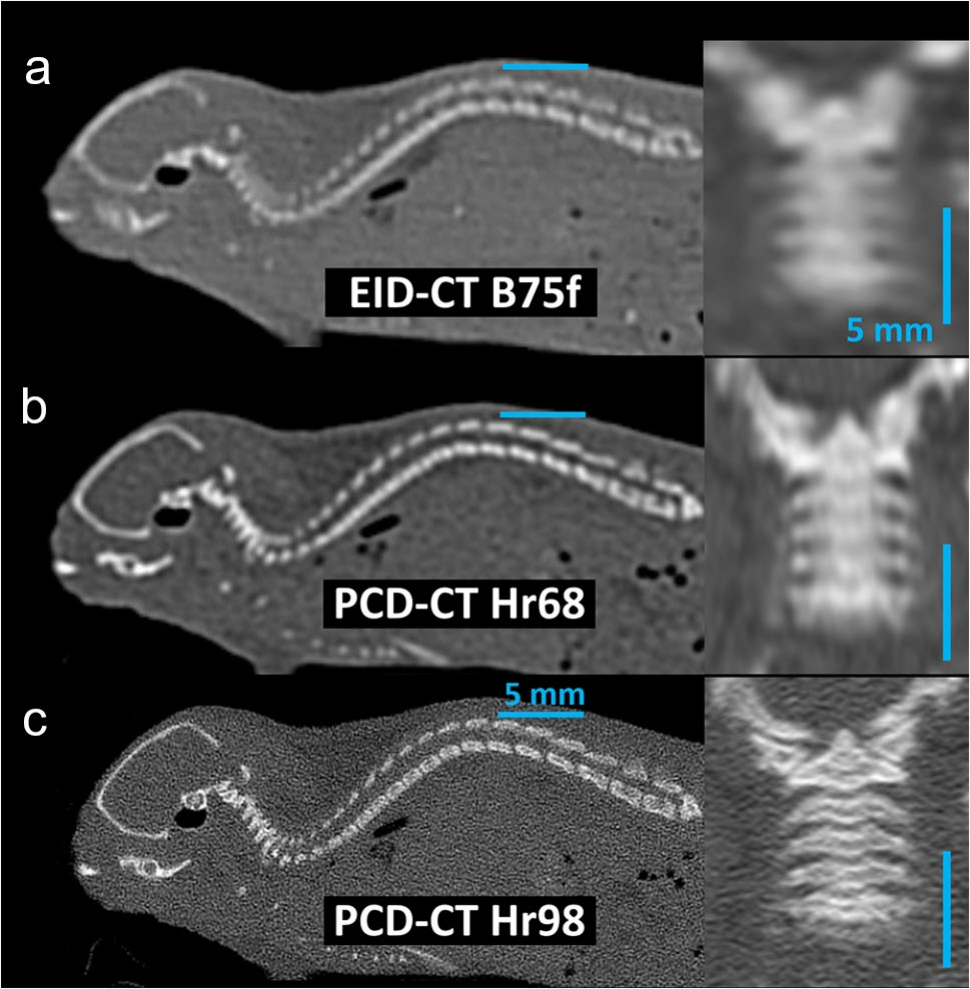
Reconstructed series used for analysis. Sagittal reconstructions of the whole spine (slice thickness: 0.2 mm) and coronal reformations of the cervical spine (slice thickness: 0.6 mm) at EID-CT B75f (a), PCD-CT Hr68 (b), and PCD-CT Hr98 (c) at CTDI_Vol_ = 15 mGy. CTDI_Vol_, volumetric CT dose index; EID-CT, energy-integrating detector CT; PCD-CT, photon-counting detector CT; B75h, Hr68, and Hr98 are abbreviations for specific reconstruction algorithms
